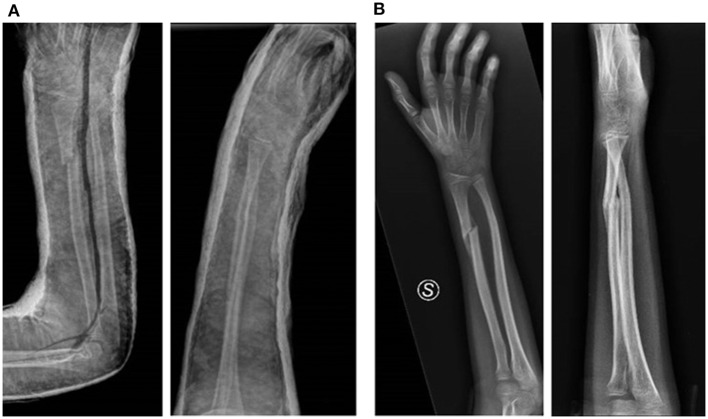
Left forearm fracture in 11-year-old obese patient, treated conservatively (A). A 1-month X-ray follow-up shows a significant loss of reduction was evident, with improved angulation of the fragments (B).
